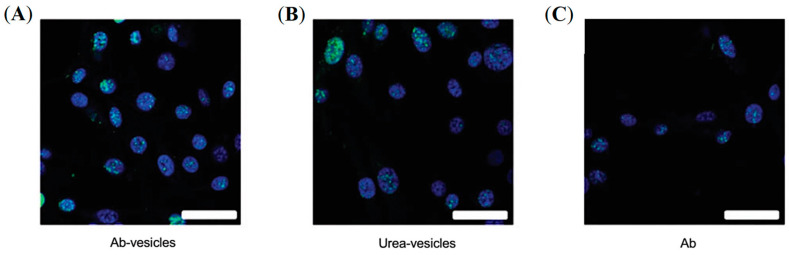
Treatment of prostate cancer using PSMA-targeted, ^225^Ac-labelled liposomes. Immunofluorescent images of g-H2AX foci (green) in cell nuclei (blue) upon treatment with Ab-targeted vesicles (A), urea-targeted vesicles (B), and radiolabeled Abs (C). Scale bar, 40 mm. Reprinted with permission from Ref [63]. Copyright 2016, American Association of Cancer Research.
